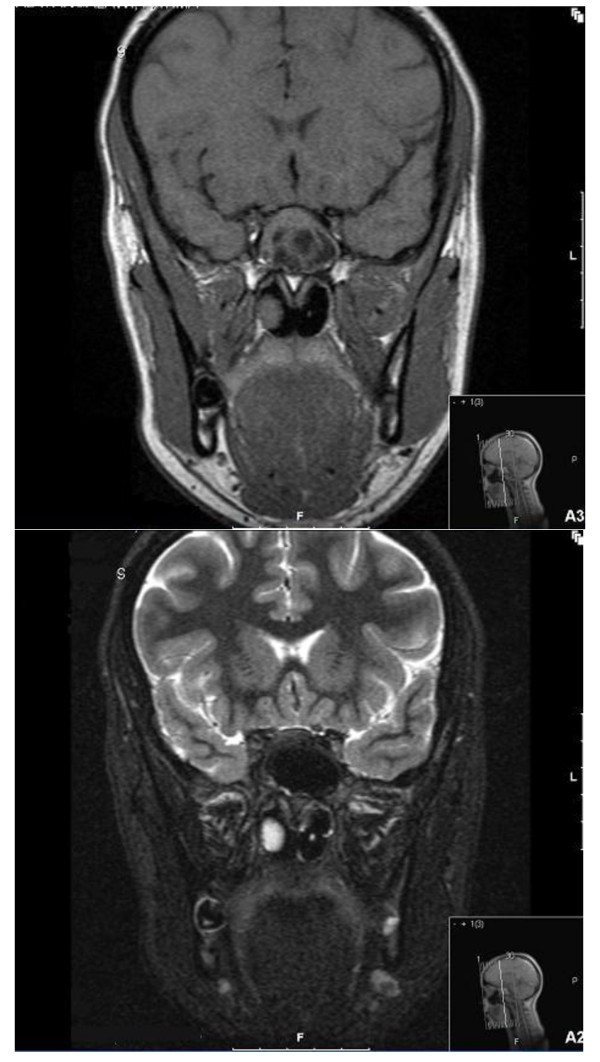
Magnetic resonance images of sinuses demonstrate a fungal infection. Coronal T1-weighted (above) and T2-weighted (below) images demonstrate enlargement of the left sphenoid sinus. The posterior aspect of the left nasal polyp and the contents of the large sphenoid sinus are of low T2 and short inversion time inversion recovery (STIR) signal intensity, indicating the presence of coexisting fungal infection.
